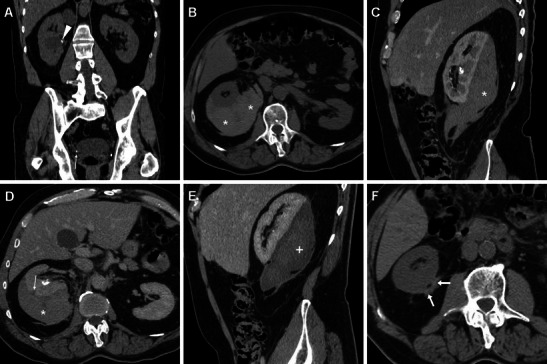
A 63-year-old male patient with comorbidities suffered from syncope, flank pain and tenderness, with a 3 g/dl haemoglobin drop 6 h after ESWL treatment of right-sided lithiasis of the renal pelvis (*arrowhead*) and distal ureter (*thin arrows*) at preoperative unenhanced MDCT (A). After bedside ultrasound (not shown) detected an inhomogeneously echoic renal collection, urgent multiphase contrast-enhanced MDCT including multiplanar reformatted images (B, C, D) confirmed a hyperattenuating (55–60 HU) acute subcapsular renal haematoma (*) extending towards the diaphragmatic crus. The ventrally displaced right kidney appeared functioning with residual lithiasis and a focal parenchymal laceration (thin arrow in D) in the nephrographic acquisition. Atelectasis and moderate pleural effusion were seen at the ipsilateral lung base. Hydronephrosis, active bleeding and urine extravasation were excluded. Conservative treatment including blood transfusions achieved clinical improvement and stabilisation of the haematocrit. Follow-up MDCT 3 weeks later (E) showed haematoma (*) with decreased attenuation (35–40 HU) indicating initial liquefaction. One year later, unenhanced MDCT during another episode of renal colic demonstrated resolved haematoma (arrows in F)
